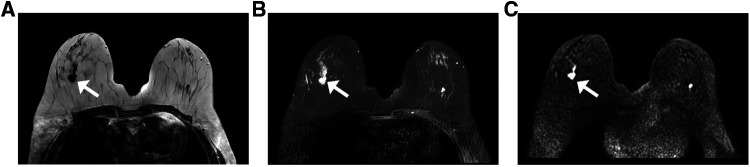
MRI from a 63-year-old woman with a palpable abnormality in the right breast the MRI shows an irregular mass with a spiculated margin in the upper outer quadrant. The tumor exhibits the following characteristics: (A) a low signal on the T1WI; (B) a high signal on the fat-suppressed T2WI; and (C) a high signal on the DWI (white arrows indicate the locations of lesions). Abbreviations: MRI, magnetic resonance imaging; T1WI, T1-weighted imaging; T2WI, T2-weighted imaging; DWI, diffusion-weighted imaging.
